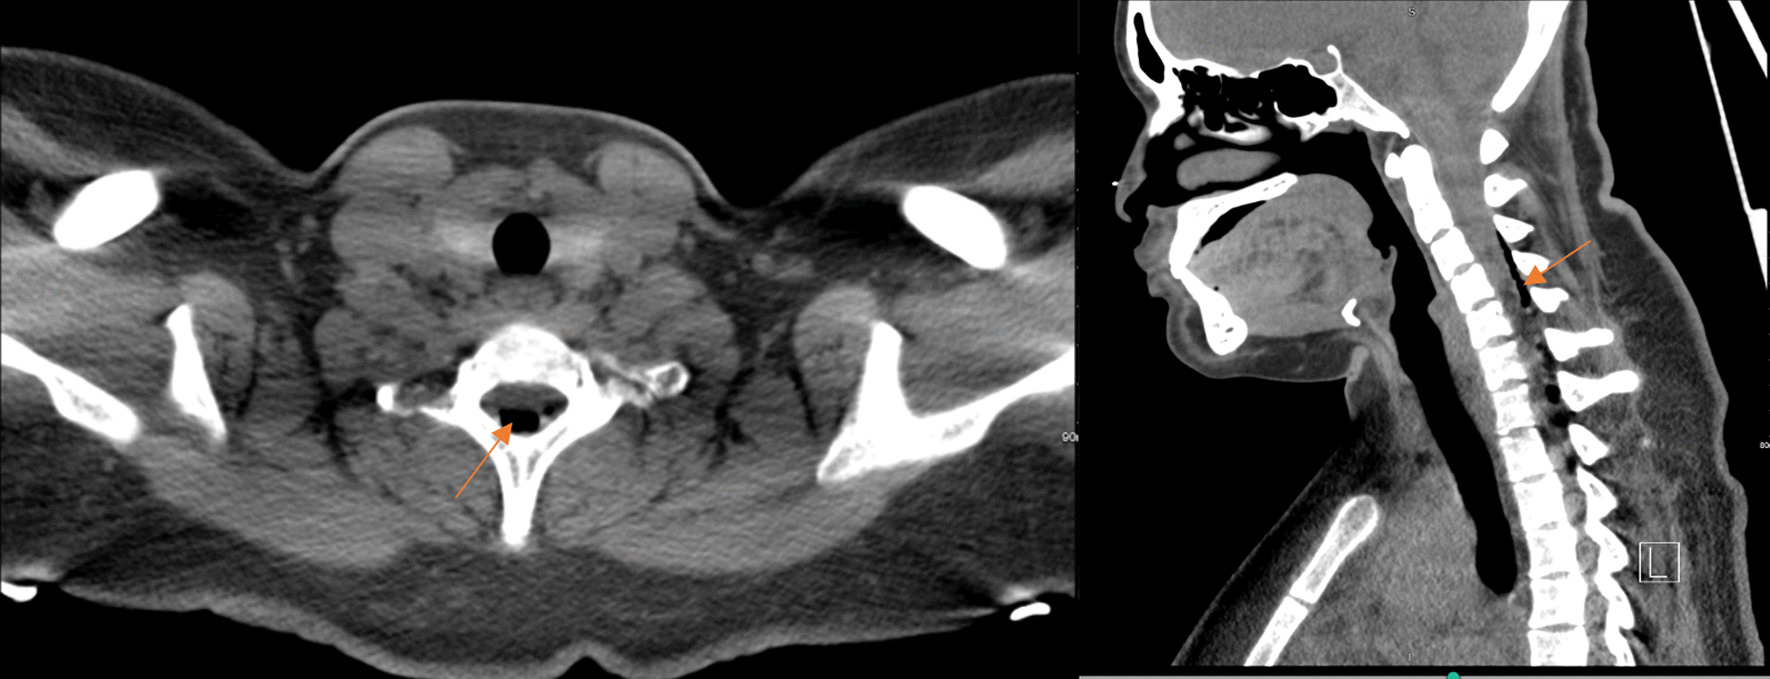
Computed tomography of the neck on the second presentation showing decreased small dorsal cervical/upper thoracic spine epidural air. A Axial view, B Sagittal view. Orange arrows identify the abnormal presence of air
